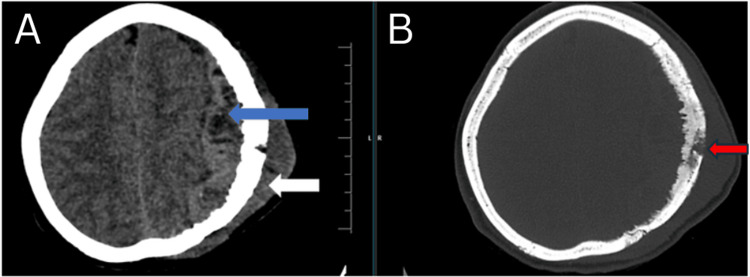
(A) Computed tomography brain plain axial section: brain window showing extra-calvarial (white arrow) and intra-calvarial, extra-axial (blue arrow) hyperdense lesion in the left frontoparietal region with areas of hypodensity within; (B) computed tomography brain plain axial section: bone window showing irregular destruction of the inner and outer table of the calvarium in the left frontoparietal region showing osteolysis (red arrow)
